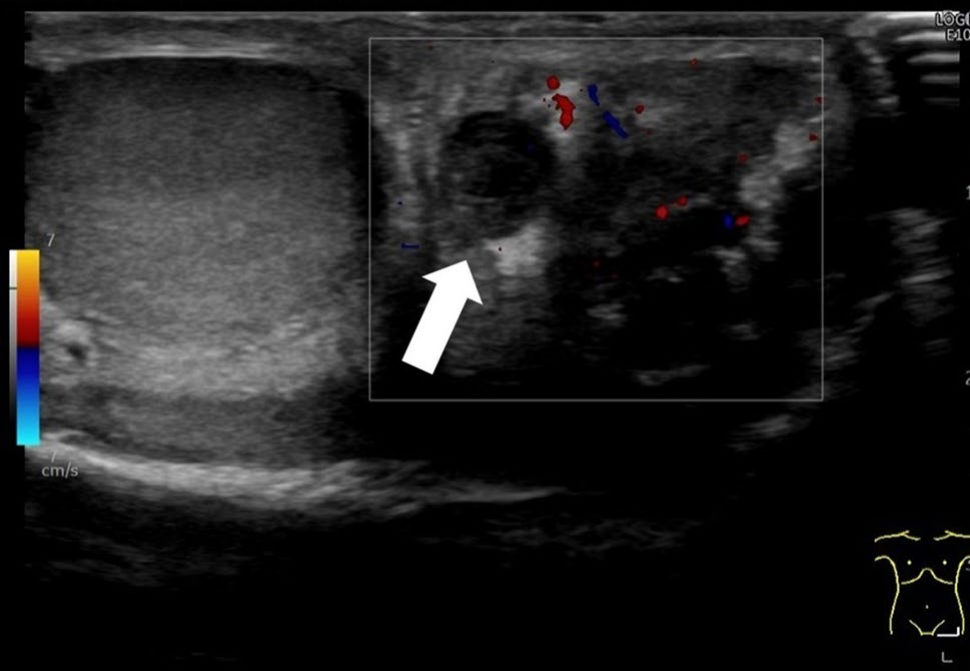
Ultrasonographic images of a 15-year-old boy with torsion of appendix epididymis. Ultrasonography shows the presence of a low-echoic mass adjacent to the testis. Color Doppler ultrasonography shows absence of blood flow within the low-echoic lesion. A diagnosis of torsion of the appendiceal testis was made
